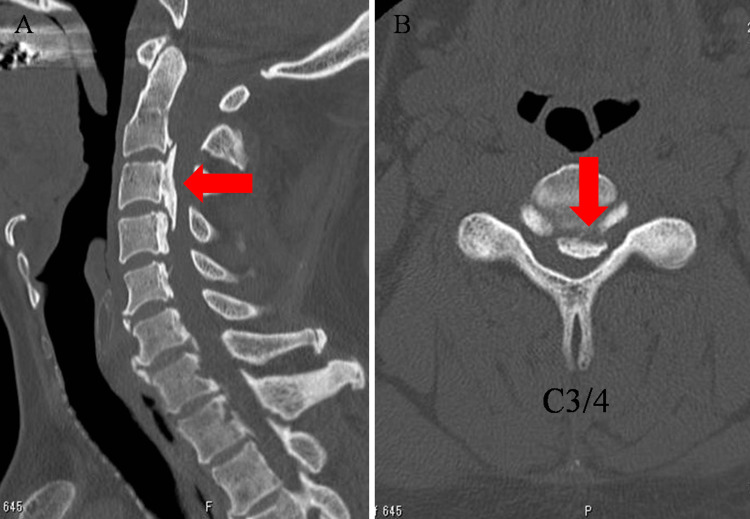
CT scans of the cervical spine at the initial examination (A) CT scan image of the midsagittal section; (B) CT scan image of the horizontal section. Ossification of the posterior longitudinal ligament is seen between C2 and C4 (red arrows).
